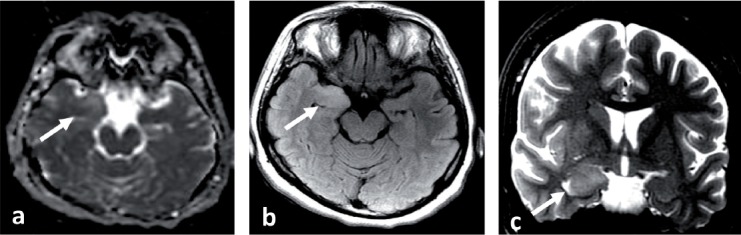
MRI brain at presentation a) Axial apparent diffusion coefficient (ADC) map, b) Axial fluid attenuated inversion recovery (FLAIR) and c) Coronal T2 spin echo (T2SE) images showing abnormal high T2 and FLAIR signal intensity and swelling with blurring of the margins of right amygdala and medial right temporal lobe cortex and increased diffusivity associated on ADC map (White arrows). No abnormal enhancement (post contrast images not shown).
